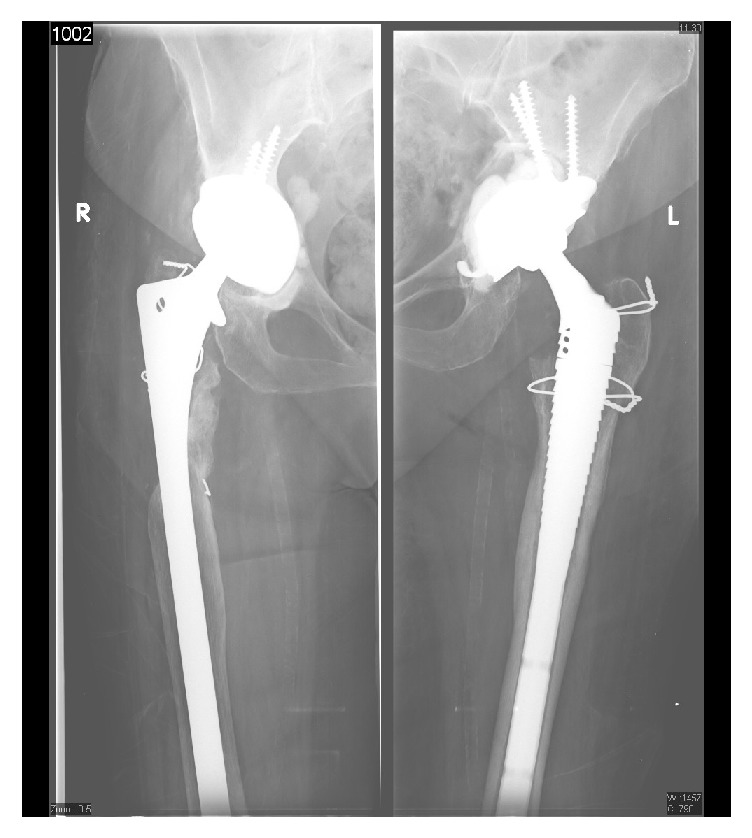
Preoperative A/P left and right hip X-rays of the patient. This figure shows an AP radiograph of the left and right hips of the patient in the immediate preoperative setting.
